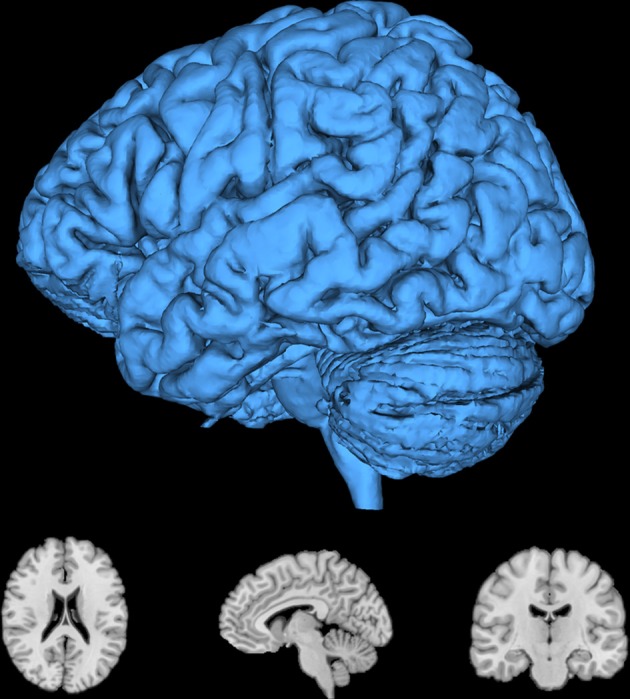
Result of the brain segmentation of the head MRI from Figure 9. A surface rendering is displayed along with axial, sagittal, and coronal slices. These images were rendered with 3D Slicer (Fedorov et al., 2012).
